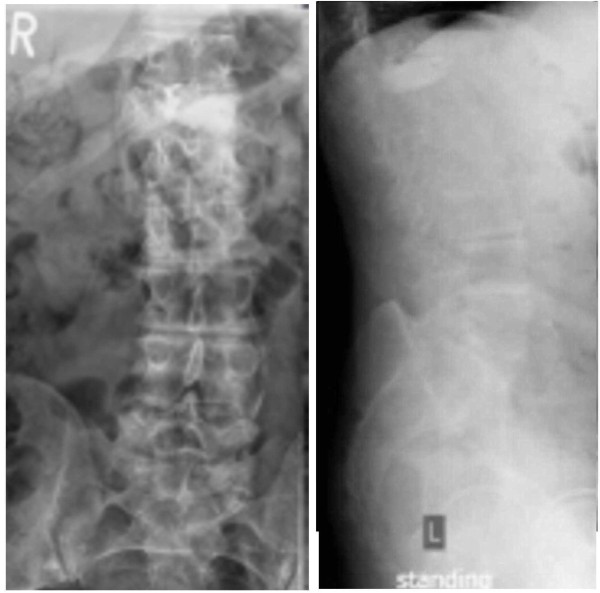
Post-kyphoplasty X-rays show anteroposterior and lateral views of the lumbar spine.
